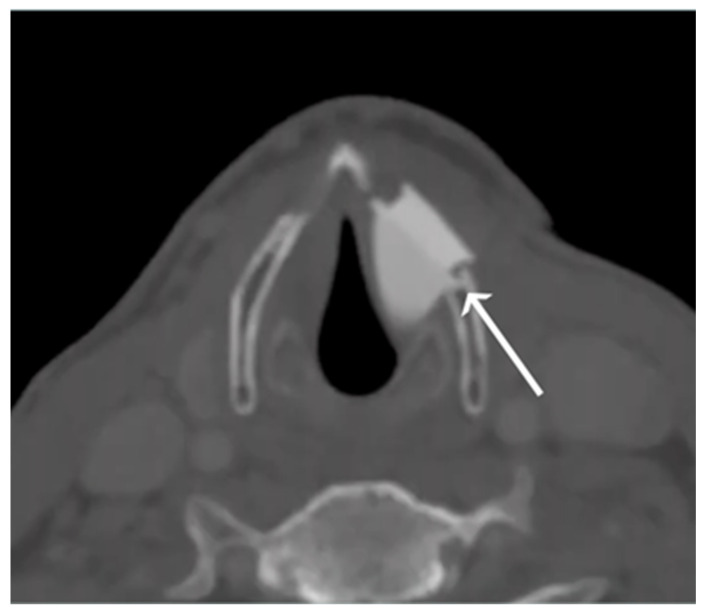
CT imaging of malpositioning of laryngeal prostheses: White arrow showing lack of proper engagement with the thyroid lamina in the posterior area.
